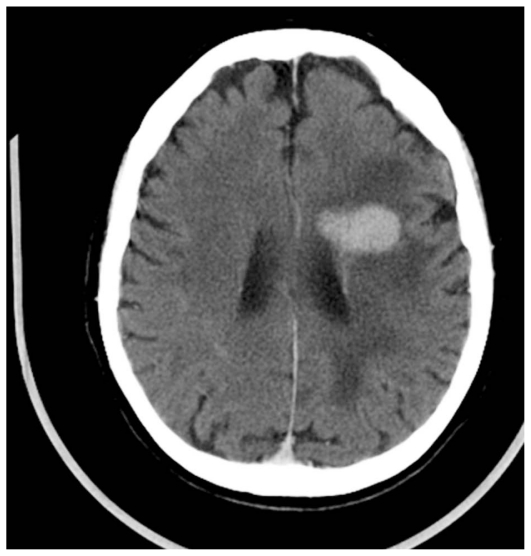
PCNSL: isolated lesion in the left brain hemisphere (homogeneous contrast enhancement on CT-scan).
